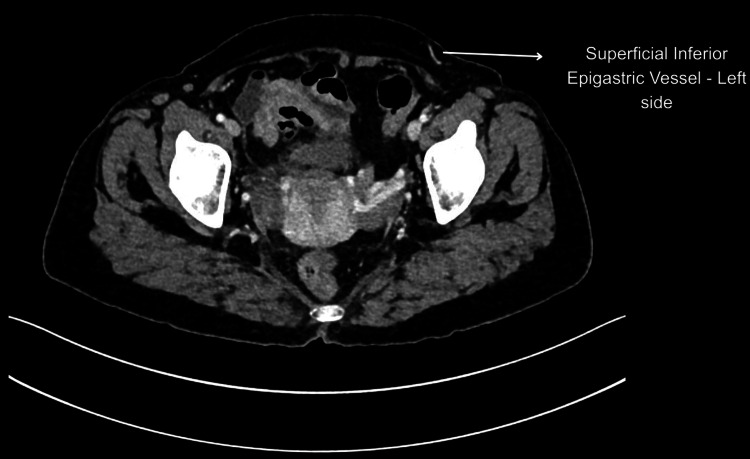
Large calibre of the superficial inferior epigastric vein on CT imaging
